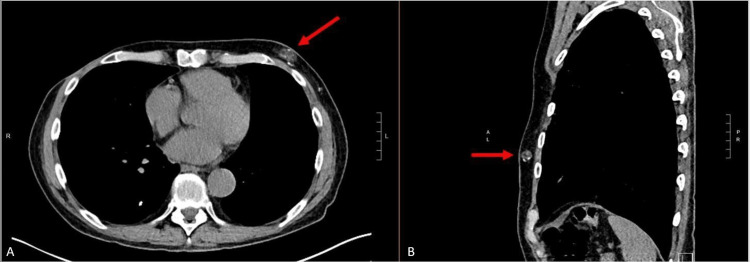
Preoperative computed tomography scan of the chest without contrast showing nodularity of the left chest wall (marked with red arrows). Left (A) shows the axial view. Right (B) shows the sagittal view.
